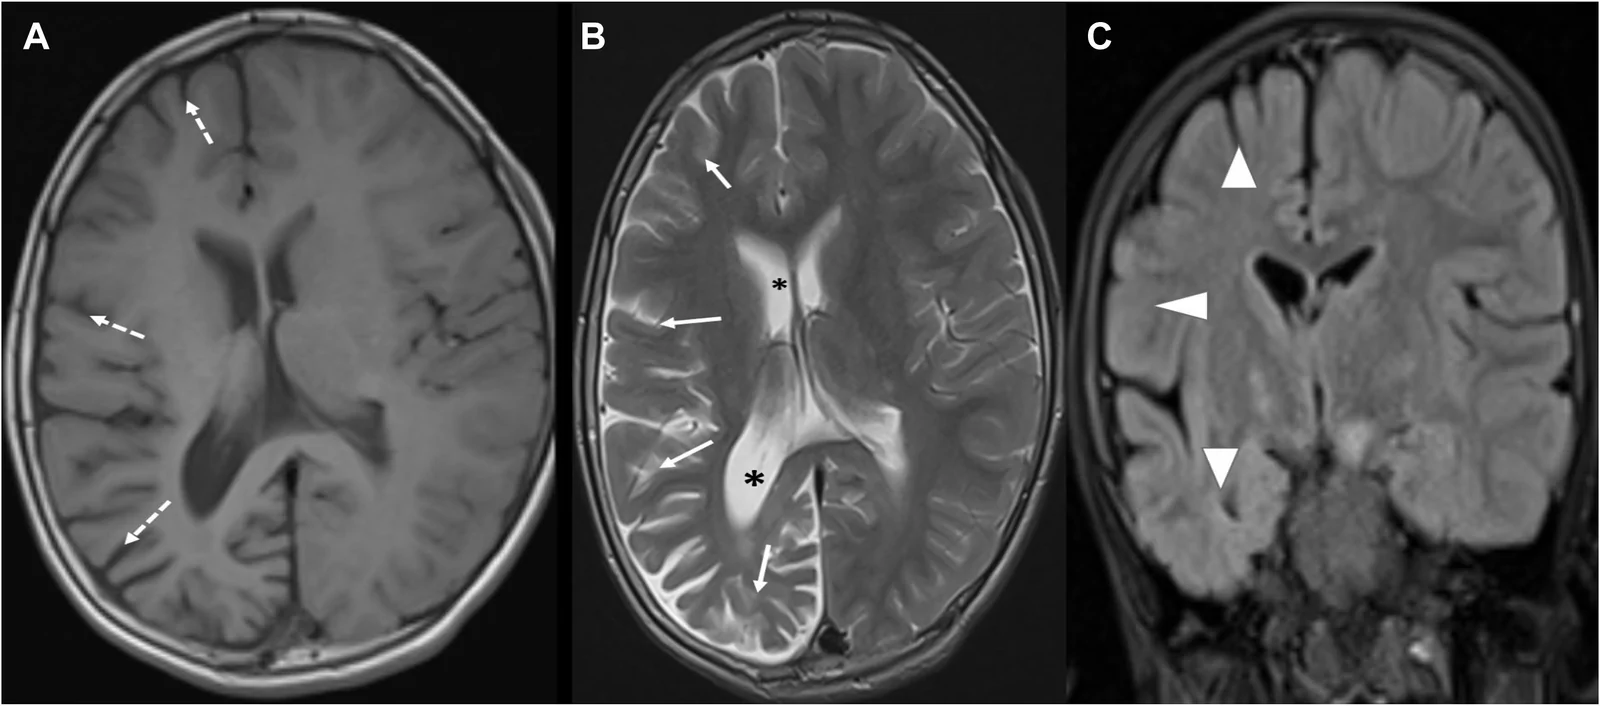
Non-contrast brain MRI shows right hemicerebral atrophy in a 6-year-old boy with NF1. (A) Axial T1-weighted image shows right cerebral hemisphere preserved gray-white matter differentiation with volume loss evidenced by asymmetrically widened sulci (*dashed white arrows*). (B) Axial T2-weighted image shows right hemispheric atrophy (*solid white arrows*) with associated ex-vacuo dilation of the extra-axial space and ipsilateral lateral ventricle (*asterisk*). (C) Coronal FLAIR shows the asymmetry in the cerebral hemispheres (*arrow heads*). *MRI,* magnetic resonance imaging; *NF1,* neurofibromatosis type 1.
